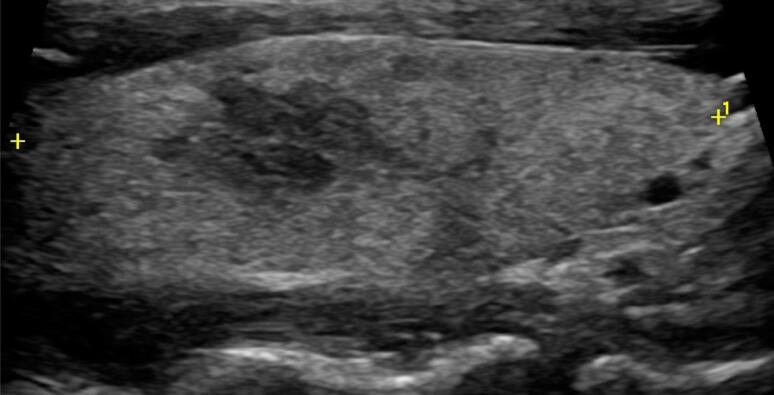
Thyroid ultrasound: in the right thyroid lobe, a 15 × 8 × 7 mm hypoechoic lesion is seen.
